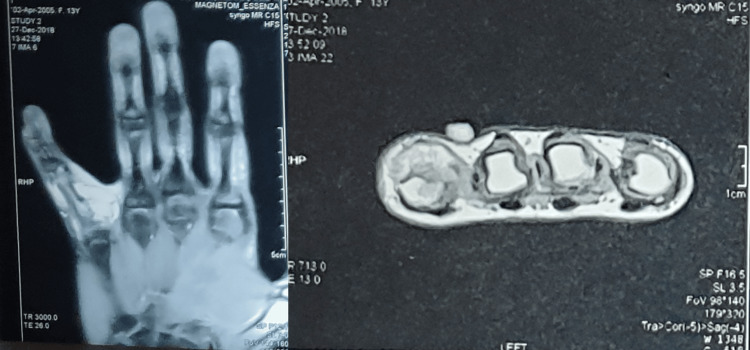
Coronal and axial views of the MRI scan of the left hand show a lesion surrounding the proximal phalanx of the little finger.
